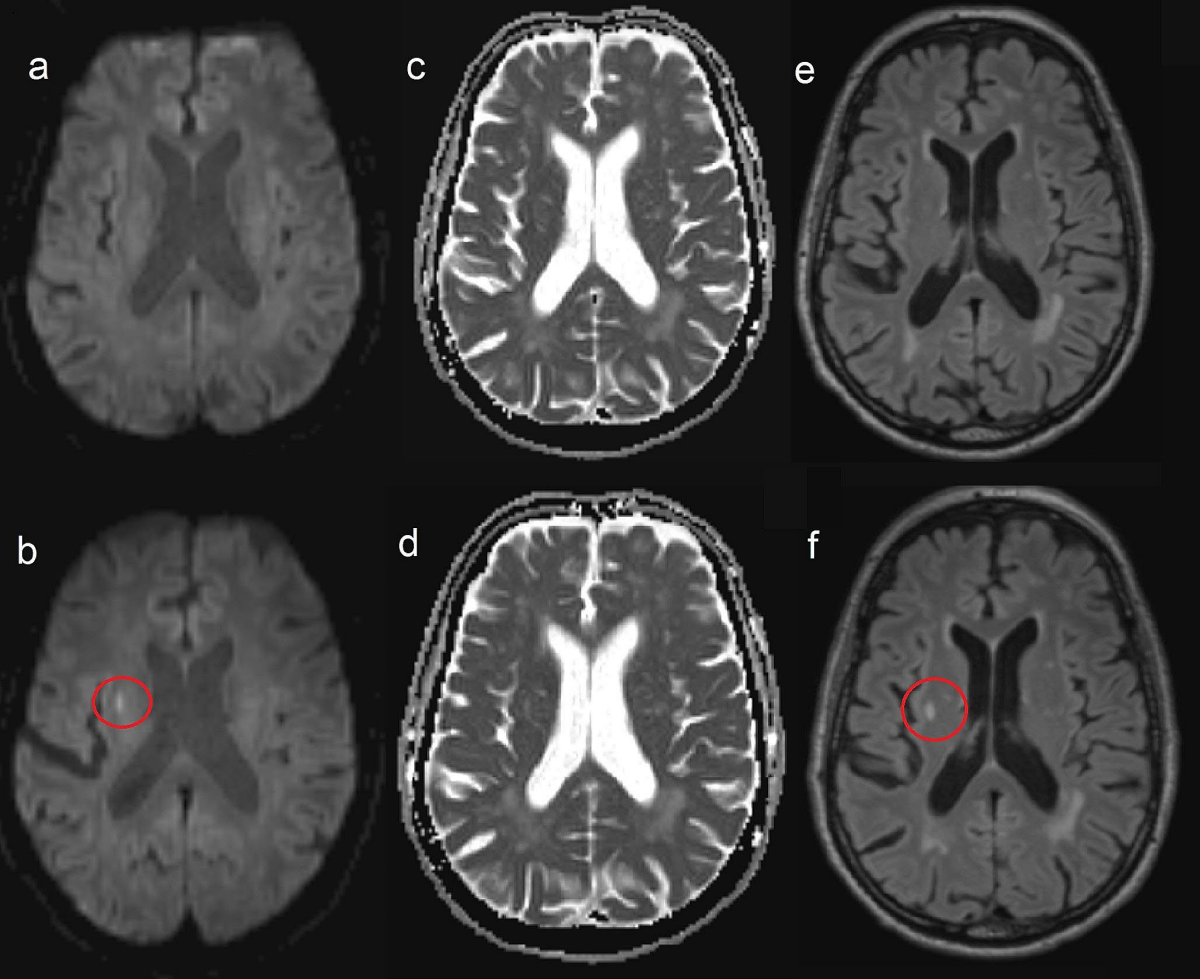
New cerebral infarction on 3-month follow-up MRI in 1 patient with COVID-19. (a) Baseline DWI-MRI scan in patient with COVID-19 (b) Follow-up DWI-MRI scan in same patient, with lacunar infarction (hyperintensity) right (red circle) (c) Baseline ADC-MRI scan in same patient (d) Follow-up ADC-MRI scan, no signs of recent ischemia (no corresponding hypointensity) (e) Baseline FLAIR MRI scan in same patient (f) Follow-up MRI showing corresponding hyperintense lesion in (a) indicating semi-recent lacunar infarction right, converted to WMH. Abbreviations: MRI = magnetic resonance imaging, COVID-19 = Coronavirus Disease 2019, DWI = diffusion-weighted imaging, ADC = apparent diffusion coefficient, FLAIR = fluid-attenuated inversion recovery, WMH = white matter hyperintensity
